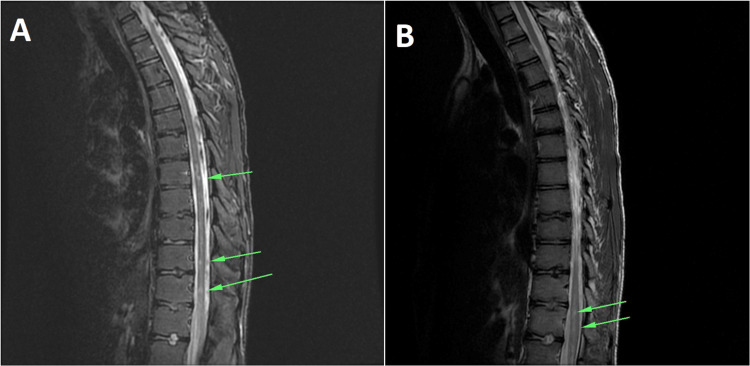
(A) T2-weighted sagittal MRI view of the thoracolumbar spine. (B) T1-weighted sagittal MRI view of the thoracolumbar spine. The arrows are depicting demyelinating lesions, which are located below the T7 vertebra level and and until the L1/L2 spine levels.
